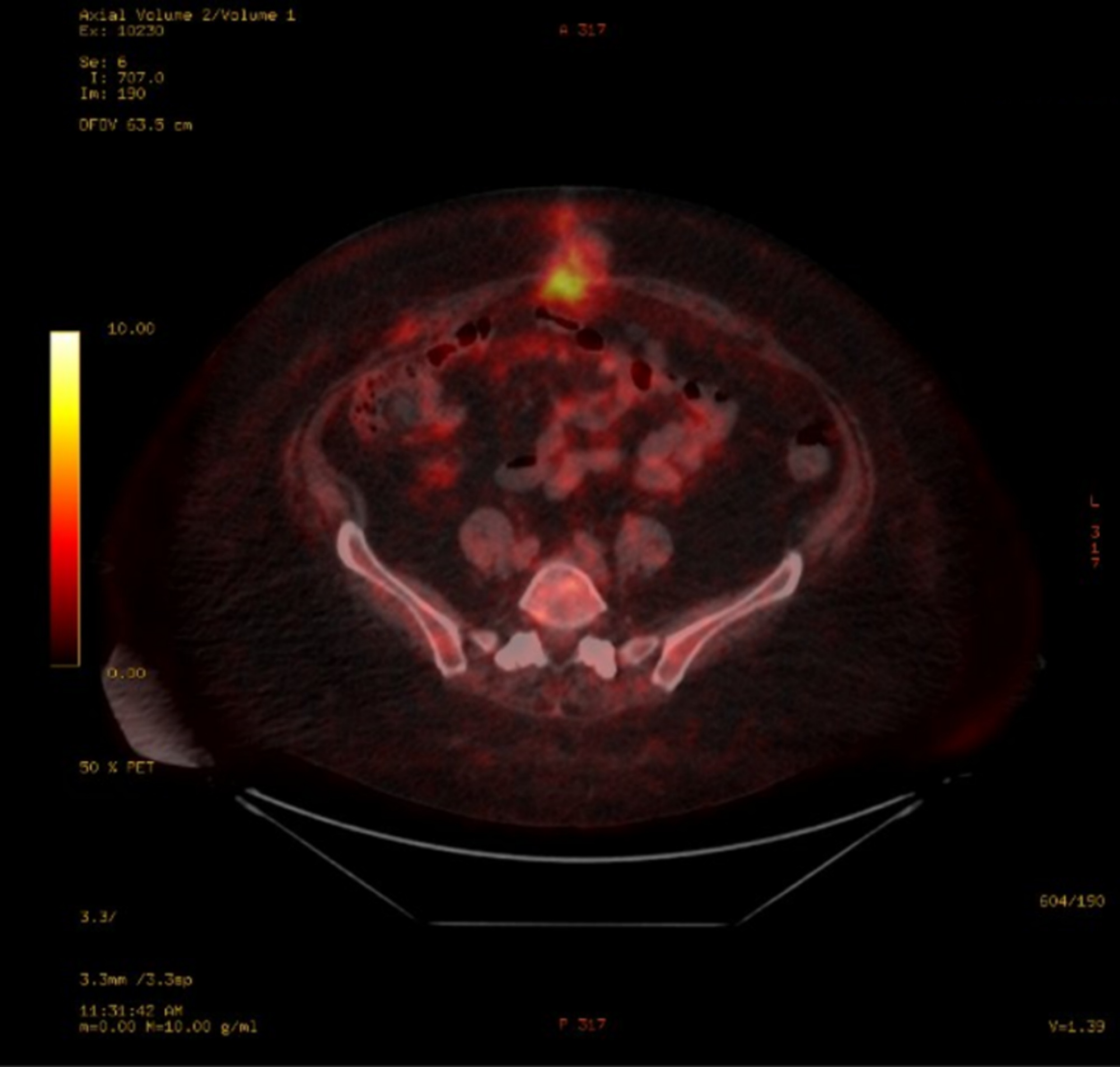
PET-CT axial - No definite evidence for metastatic disease. Patient is status post appendectomy. PET-CT: positron emission tomography-computed tomography
